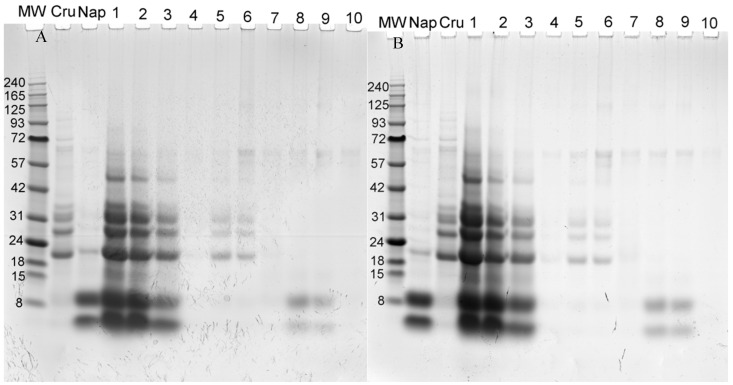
Electrophoresis pattern of fractions from conventional (A); or ultrasound-assisted (B) extraction under reducing conditions. MW molecular weight markers in kDa: (Nap) Napin standard, (Cru) Cruciferin standard, (1) extract; (2) filtered extract; (3) depigmented extract; (4) Superdex 75 peak 1 Aggregates; (5) and (6) Superdex 75 peak 2 Cruciferin; (7) Superdex 75 peak 3; (8) and (9) Superdex 75 peak 4 Napin; (10) Superdex 75 peak 5.
